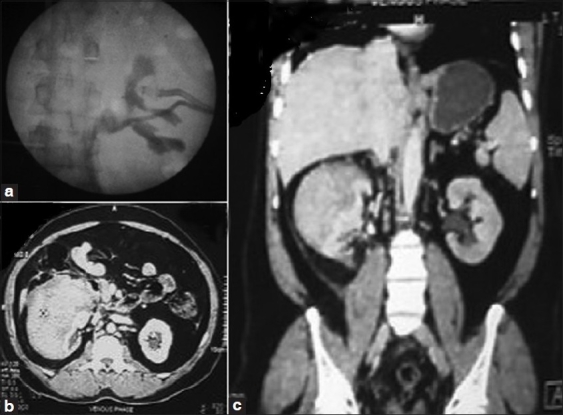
(a) Retrograde pyelogram (RGP) showing stretched out Pelvicalyceal system (PCS) with drain *insitu* in hematoma. (b,c) CT images showing subcapsular hematoma with compressed and displaced right renal parenchyma
